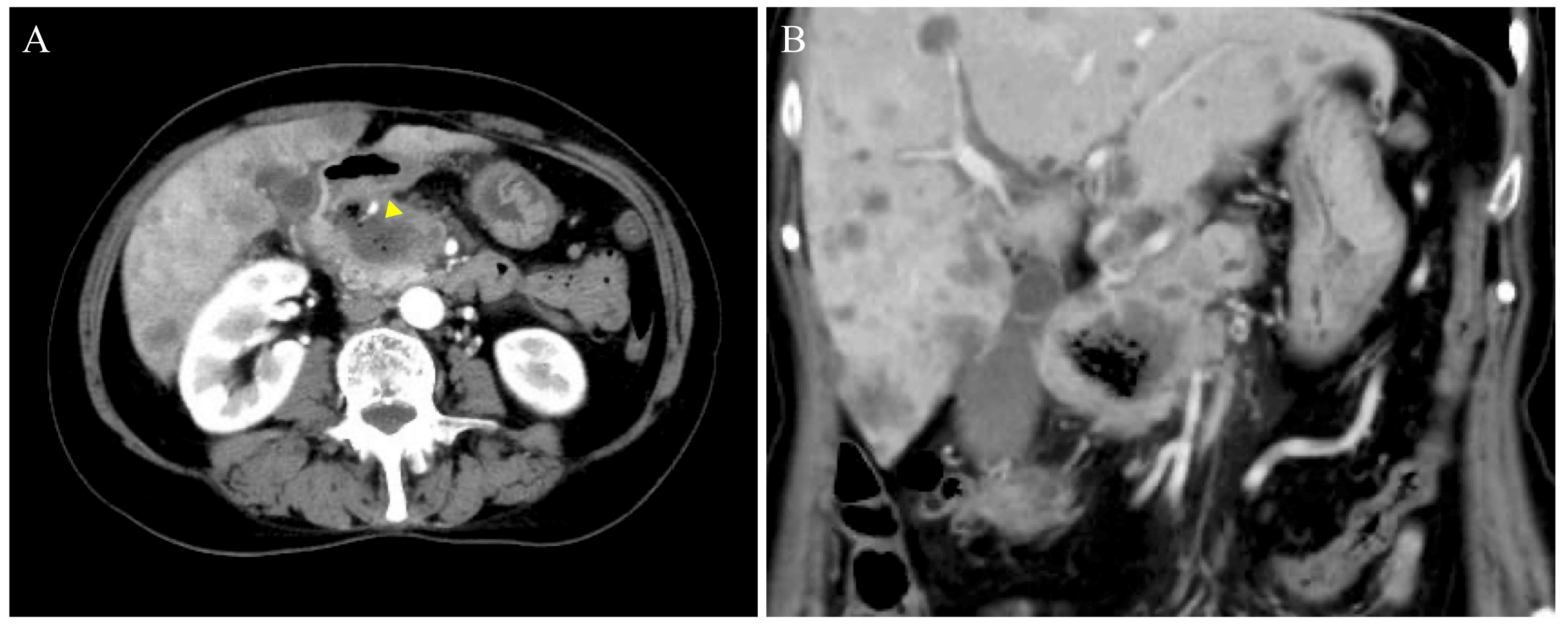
Contrast-enhanced CT of the abdomen Contrast-enhanced computed tomography (CT) of the abdomen revealing pancreatic cancer from the descending duodenal leg to the pancreatic head (A) and duodenal invasion (B). Exposure of the pancreaticoduodenal arcade into the duodenum can be suspected (yellow arrowheads).
